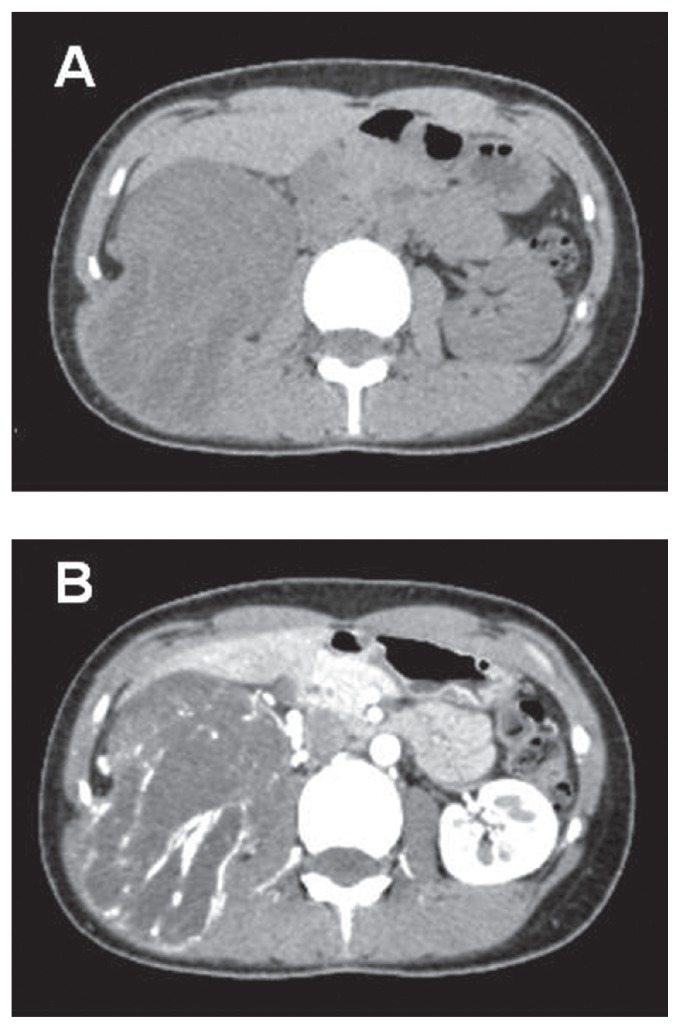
A) Abdominal computed tomography demonstrated the presence of a solid encapsulated mass with nonuniform internal structure about 11 × 9 cm in diameter, compressing the right kidney to the caudal side; B) contrast-enhanced CT showed a tumor with early enhancement in mainly dorsal side of the tumor.
